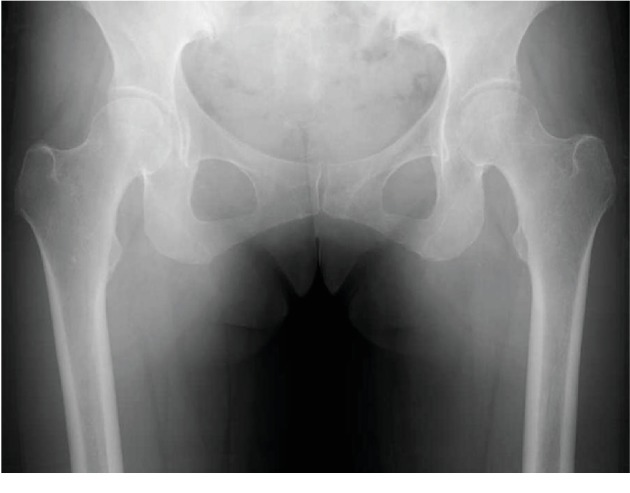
Radiograph of the hip 10 months after TKA.
